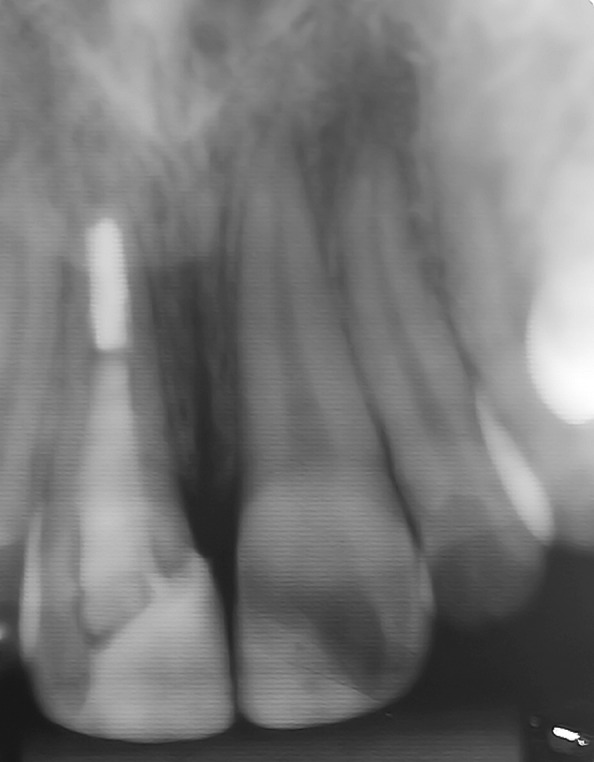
Final radiograph after aesthetic restoration
